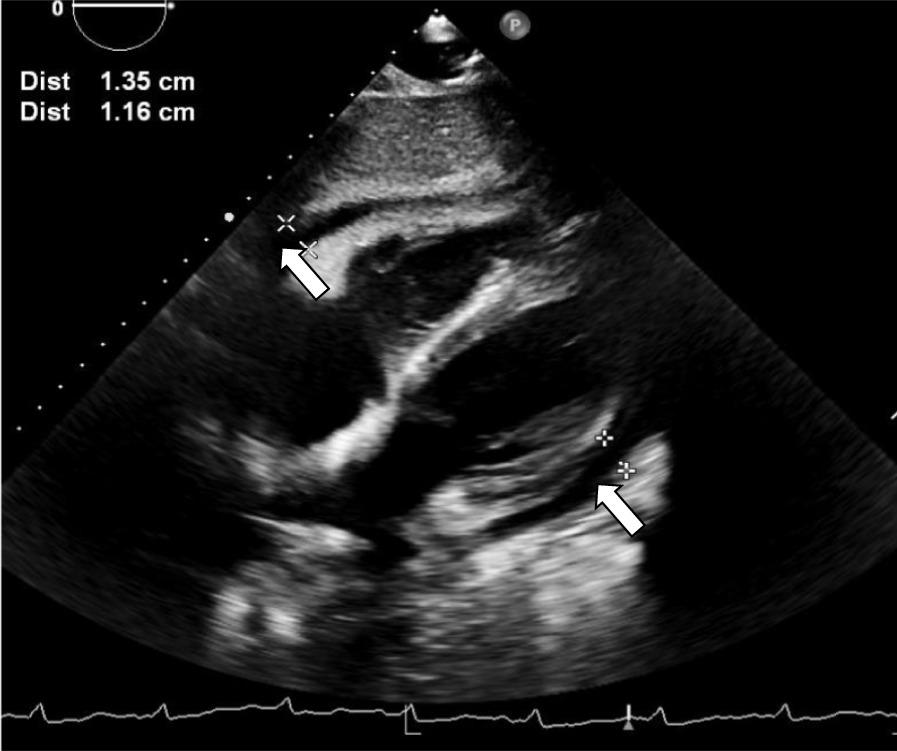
An apical four-chamber view on transthoracic echocardiogram obtained on the day of the patient’s cardiopulmonary decline (HD #6) demonstrating a moderate-sized pericardial effusion (white arrows)
